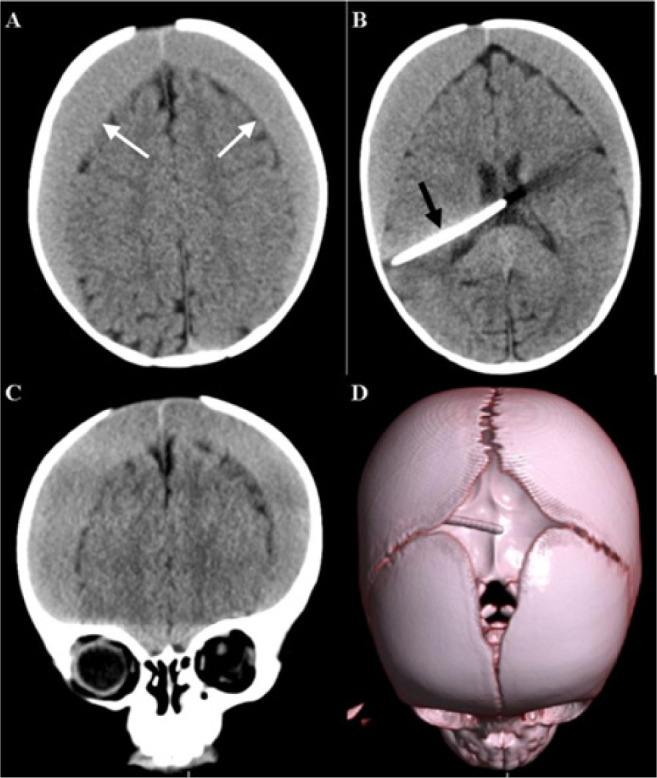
CT Scan Images A: Bilateral subdural hematoma (white arrows) B: VP shunt in situ (black arrow) C: Coronal view D: 3D reconstruction showing open fontanelles and widened sagittal suture
